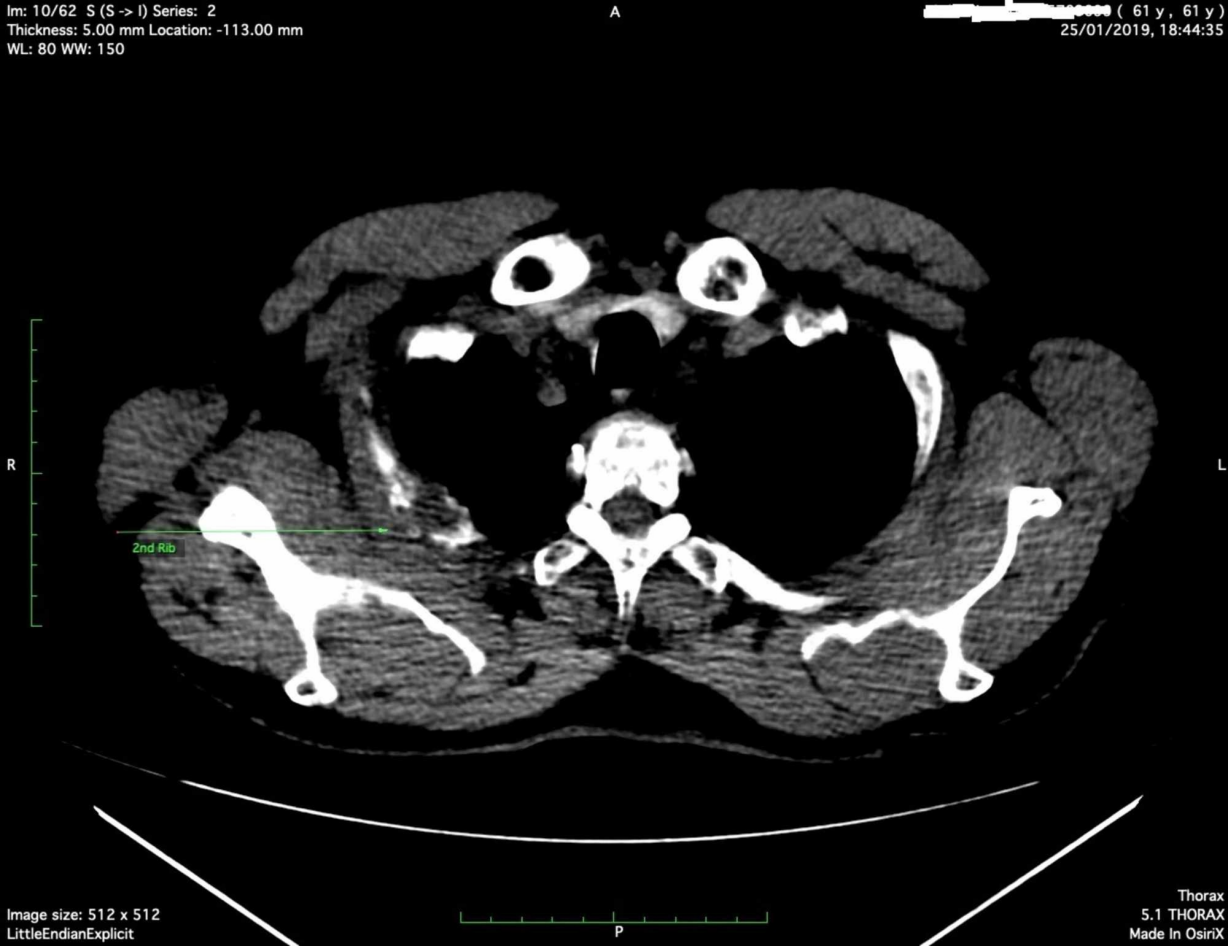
Sagital view of CT thorax showing an osteolytic area in the second rib with a surrounding mass
